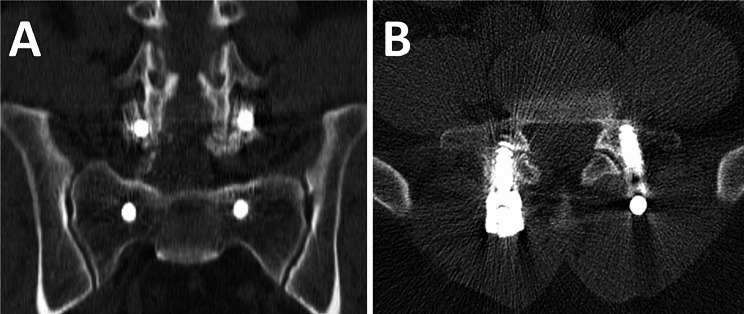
Coronal view (A) and axial view (B) of CT showing L5 right facet joint violation in a patient undergoing MIS TLIF
